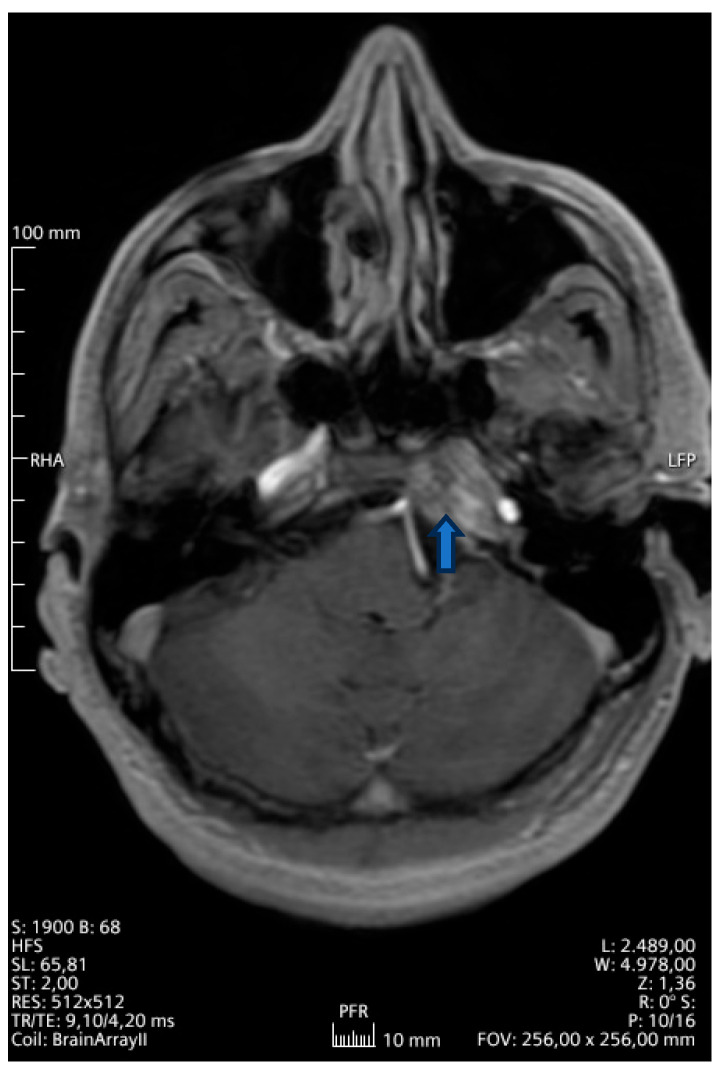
Initial MRI of the brain (T1 sequence gadolinium-enhanced) showing a lesion on the left cranial base (arrow) medially to the petrosal segment of the carotid and growing into the cavernous sinus.
